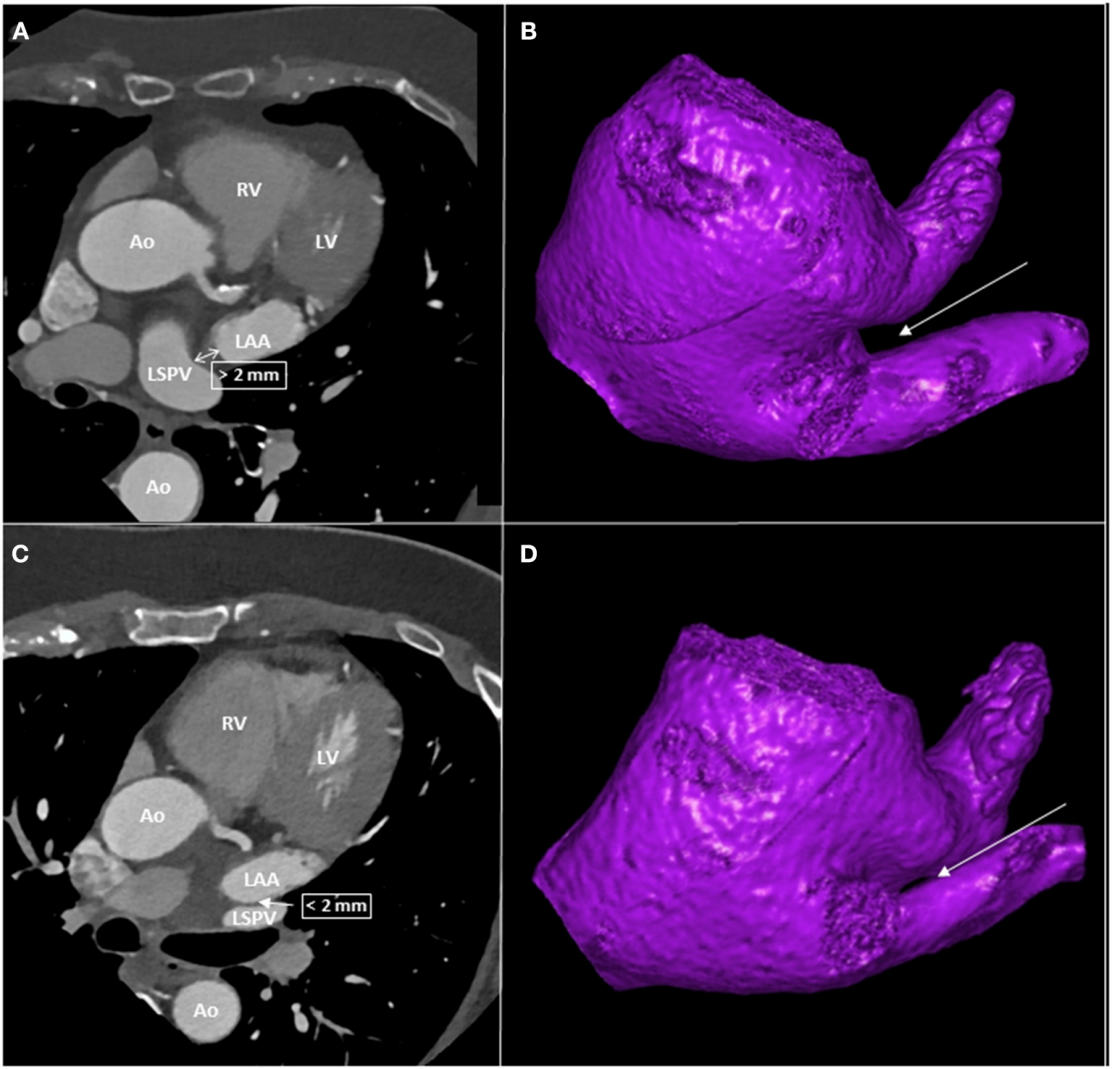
CT images of patients with and without abutting LAA-LSPV. (A) left atrial CT of a patient with non-abutting LAA-LSPV. (B) three-dimensional reconstruction of left atrium from caudal view, with a highlight on non-abutting LAA-LSPV. The LIPV was removed to give a better visualization of the LSPV. (C) left atrial CT of a patient with abutting LAA-LSPV. (D) three-dimensional reconstruction of left atrium from caudal view, with a highlight on abutting LAA-LSPV. The LIPV was removed to give a better visualization of LSPV. The arrows indicate the site of distance measurement between LAA and LSPV. Ao, aorta, CT, computed tomography, LAA, left atrial appendage, LV, left ventricle, LIPV, left inferior pulmonary vein, LSPV, left superior pulmonary vein, RV, right ventricle.
